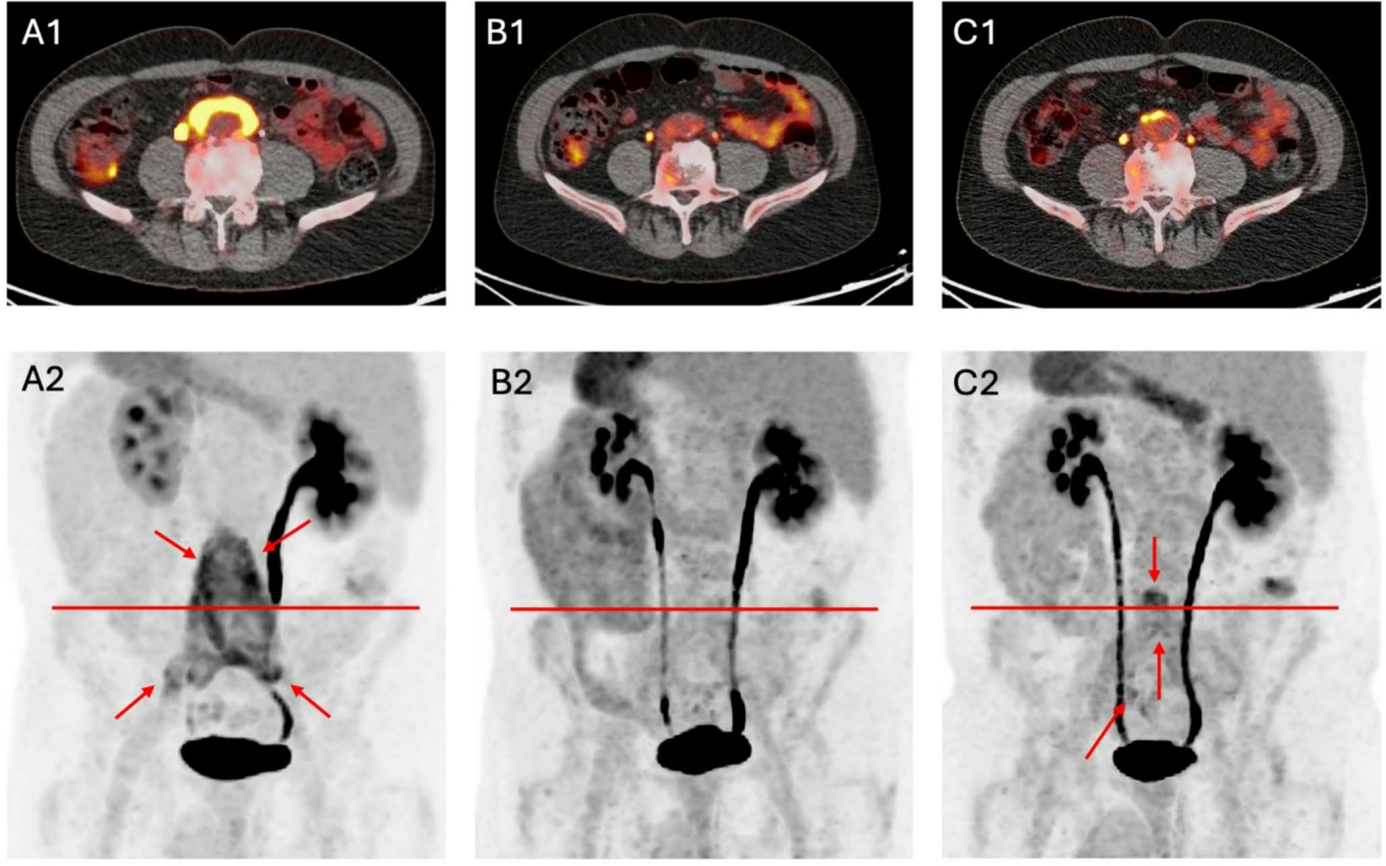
Course of retroperitoneal fibrosis in a patient showing progression between first and second follow-up. A1, B1, and C1 display axial 18 F-FDG PET/CT fusion images, with corresponding red lines in the maximum intensity projection (MIP) images (A2, B2, and C2). Red arrows delineate findings in A2 and C2. The corresponding PET values at baseline, first and second follow-up are as follows: SUV_max_: 11.9 vs. 3.5 vs. 7.5, SUV_mean_: 4.4 vs. 2.8 vs. 2.9, SUV_peak_: 9.1 vs. 2.6 vs. 4.5, and metabolic active volume (MAV): 180.5 vs. 4.6 vs. 67.7 milliliters
